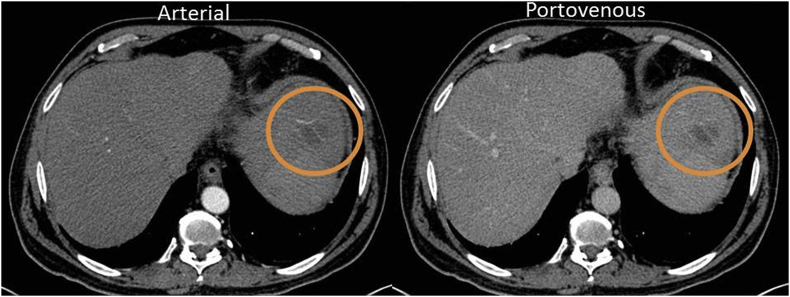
Axial CT images arterial and Porto-venous phases showing segment II non-enhancing lesion with vessels traversing through the lesion with no attenuation or occlusion (orange circles). (For interpretation of the references to colour in this figure legend, the reader is referred to the Web version of this article.)
